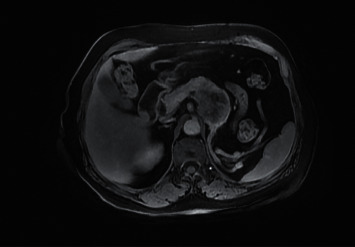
MRI T2 images of a heterogeneous hypersignal corporeal pancreatic mass.
